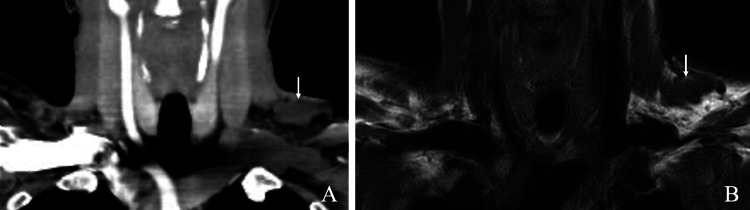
Plain computer tomography and magnetic resonance image. Plain computer tomography (A) and magnetic resonance image (B) of the neck show the anomalous muscle on the left brachial plexus (white arrow). It is not present on the right side.
